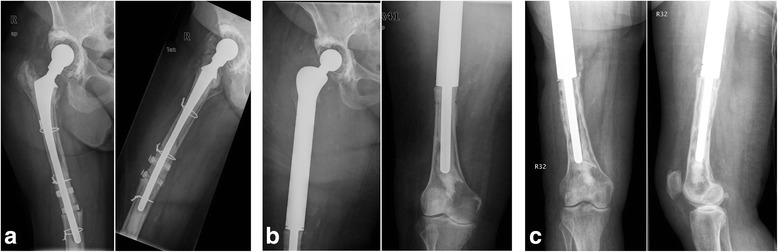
Anterior-posterior and lateral X-rays of the right hip with thigh of a 78-year-old woman with a a total hip arthroplasty (THA) after multiple revisions and acute periprosthetic fracture with implant loosening b after implantation of a proximal femur replacement and intraoperative detection of staphylococci with the clinical presentation of a PJI with implant-preserved revision and antibiosis c after 14 months, a chronic infection with increasing loosening of the implants
